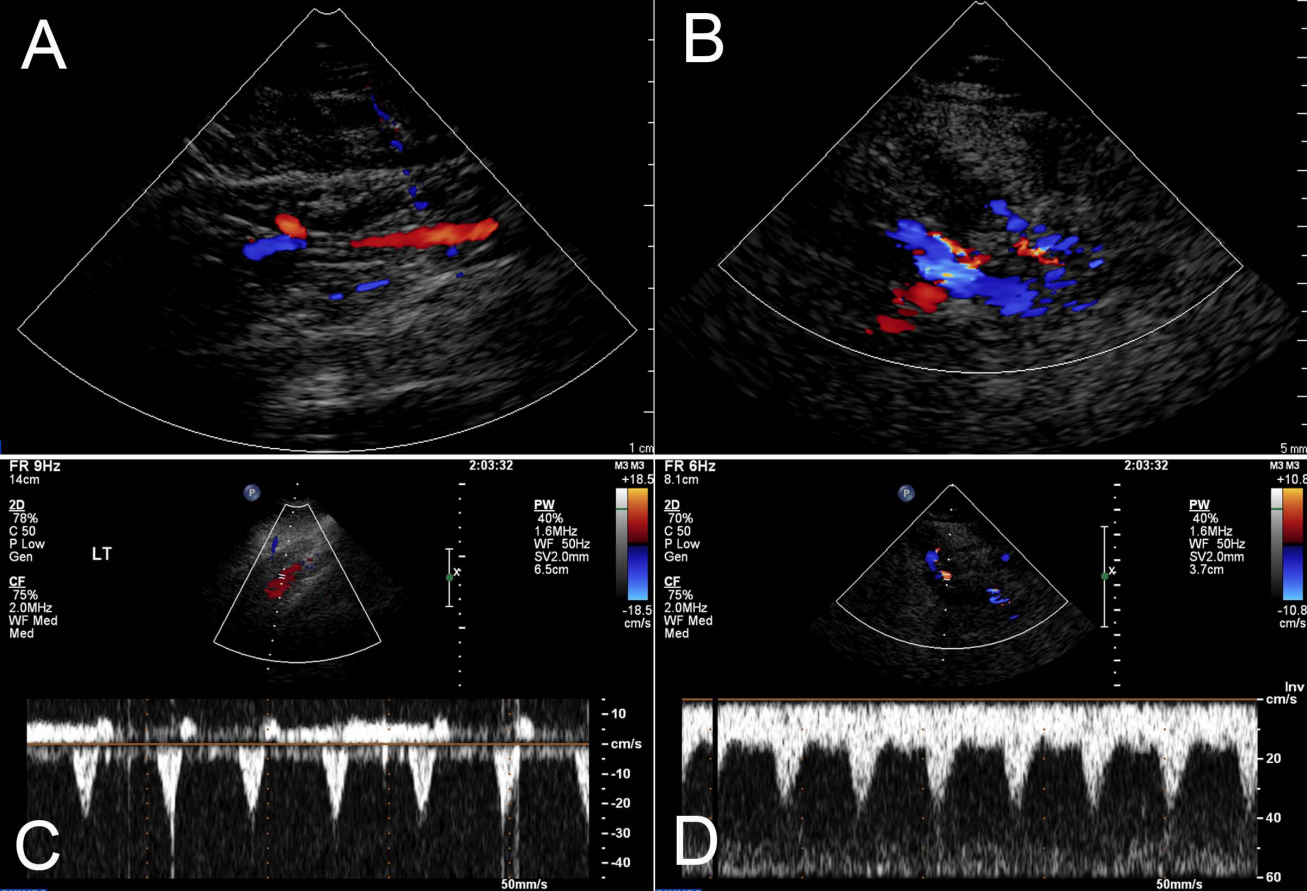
Contrast-enhanced and color Doppler ultrasound studies. Two-dimensional ultrasound images were obtained at the level of 3-5 cm below the popliteal fossa (PF). The color Doppler studies demonstrated no abnormal vasculature in the same location on the unaffected leg (A), but multiple tortuous vessel structures with pulsatile flow compatible with venous malformation fed by arteriovenous shunts (B). Pulse Doppler studies identified three specific foci of arteriovenous shunts characterized by low-resistance flow, composed of a normal venous waveform with superimposed arterial pulsatile flow (C). The same studies in the unaffected leg demonstrated a normal arterial wave form (D).
